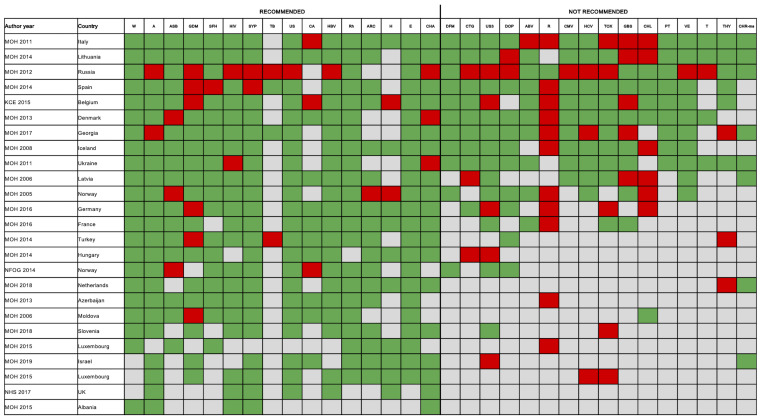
Concordance between national ANC guidelines identified by the systematic review and reference recommendations. Legend: green − in-line; red − not in-line; grey − not covered. Abbreviations: UK − United Kingdom, W − weight, A − anaemia, ASB − asymptomatic bacteriuria, GDM − gestational diabetes mellitus, SFH − fetal growth, HIV − human immunodeficiency virus, SYP − syphilis, TB − tuberculosis, US − gestation age, multiple pregnancies and fetal anomalies ultrasound, CA – cardiac anomalies, HBV – hepatitis B virus, Rh − alloimmunization, ARC − atypical red-cell alloantibodies, H − haemoglobinopathies, E – pre-eclampsia, CHA − Chromosomal abnormalities, DFM − daily fetal movement counting, CTG – antenatal cardiotocography, US3 − fetal growth, DOP − Doppler ultrasound of fetal blood vessels, ABV − asymptomatic bacterial vaginosis, R – rubella, CMV − cytomegalovirus, HCV – hepatitis C virus, TOX − toxoplasmosis, GBS − group B streptococcus, CHL − *Chlamydia trachomatis*, PT - pre-term labour, VE − vaginal examination, T − thrombophilia, THY − thyroid function, CHA-ms − chromosomal abnormalities by morphological scan.
